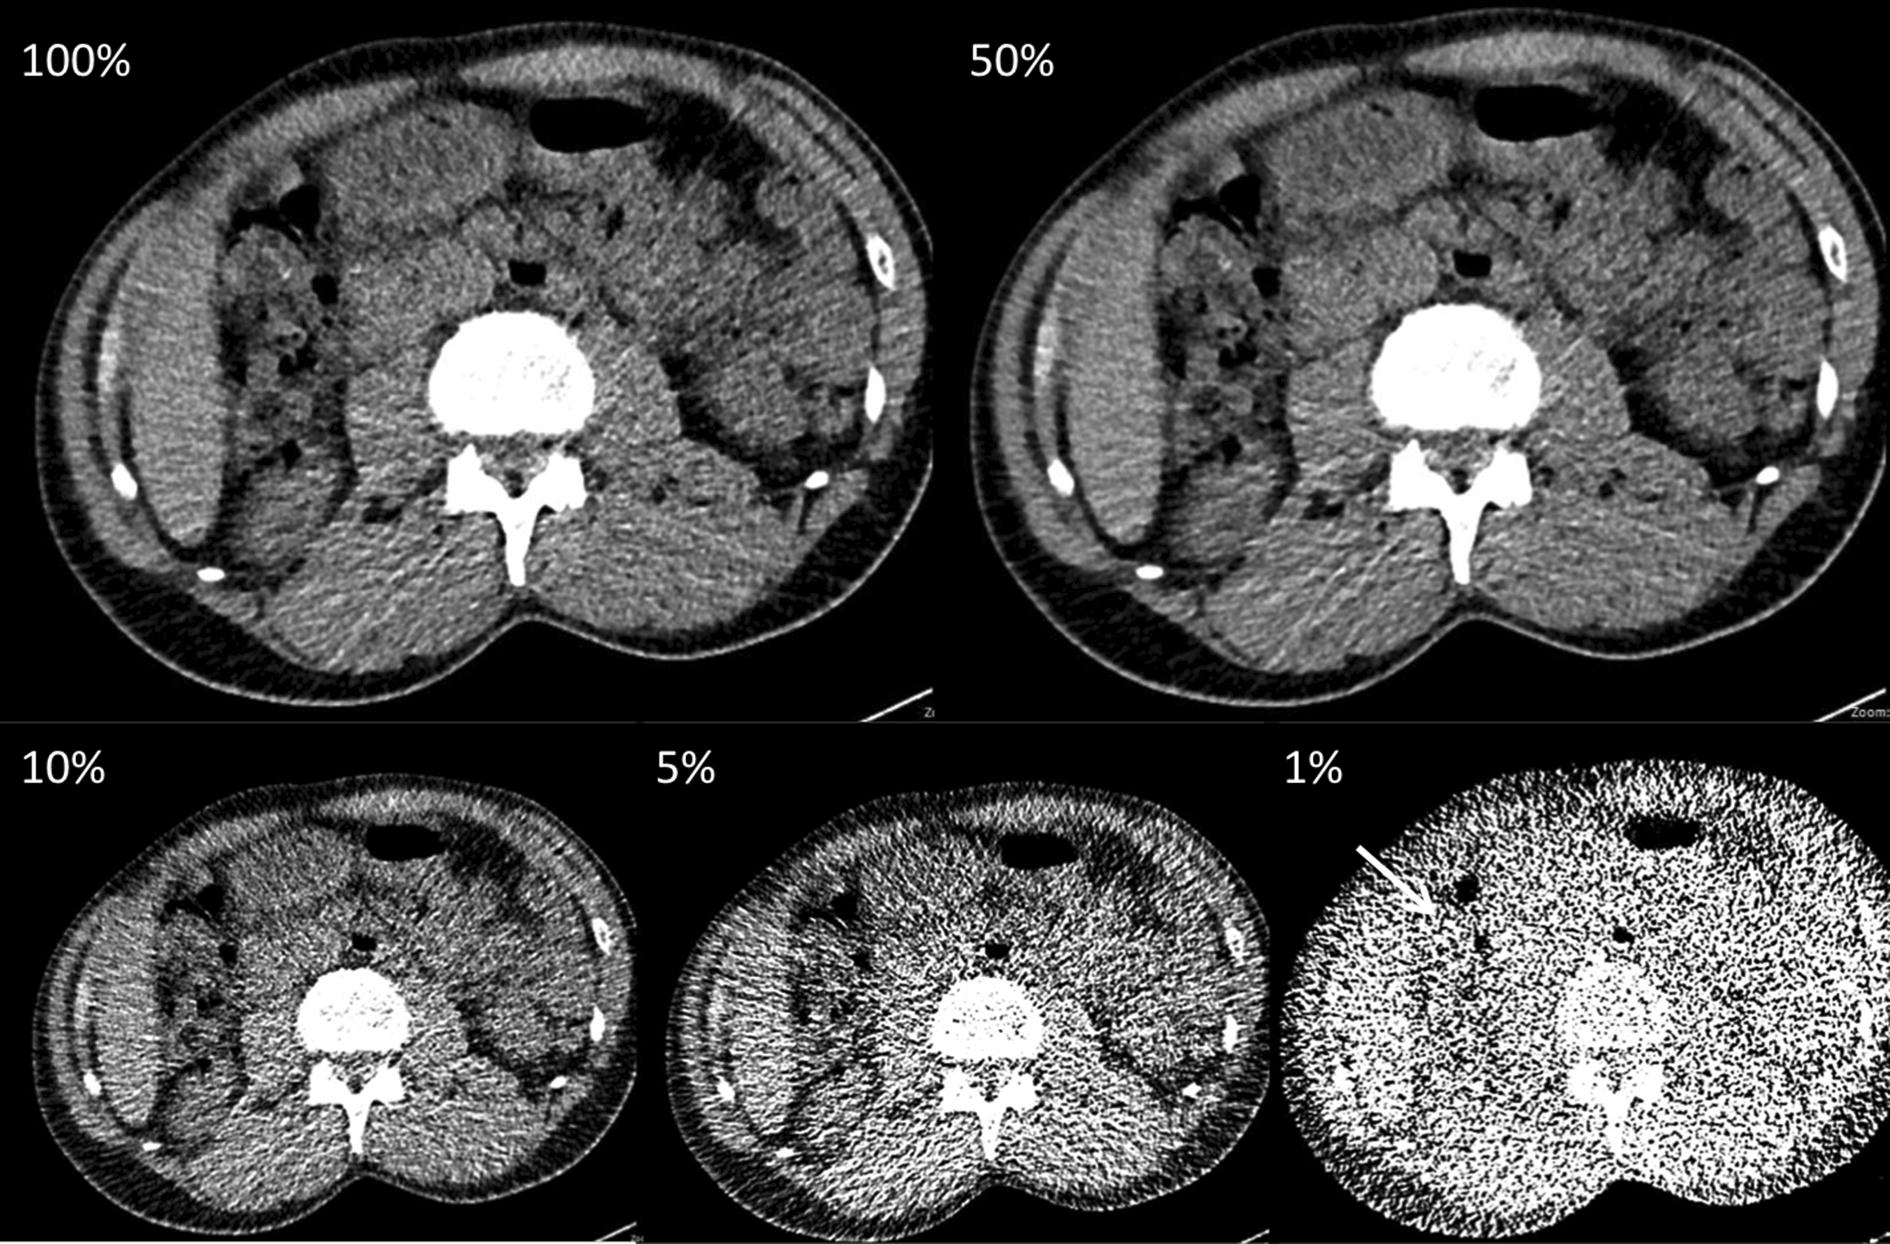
A 58-year-old individual suspected of body packing. CT examination was performed at 80 kVp with ATCM (tube current time product: 81 mAs, dose level 100% effective dose at 100%: 1.09 mSv). Axial CT scans (soft tissue window, window level 40/300) with stepwise dose reduction (100%, 50%, 10%, 5%, and 1%). In this case at 1% of initial dose (0.02 mSv), one additional false-positive solid body packet was rated by reader one (white arrow)
